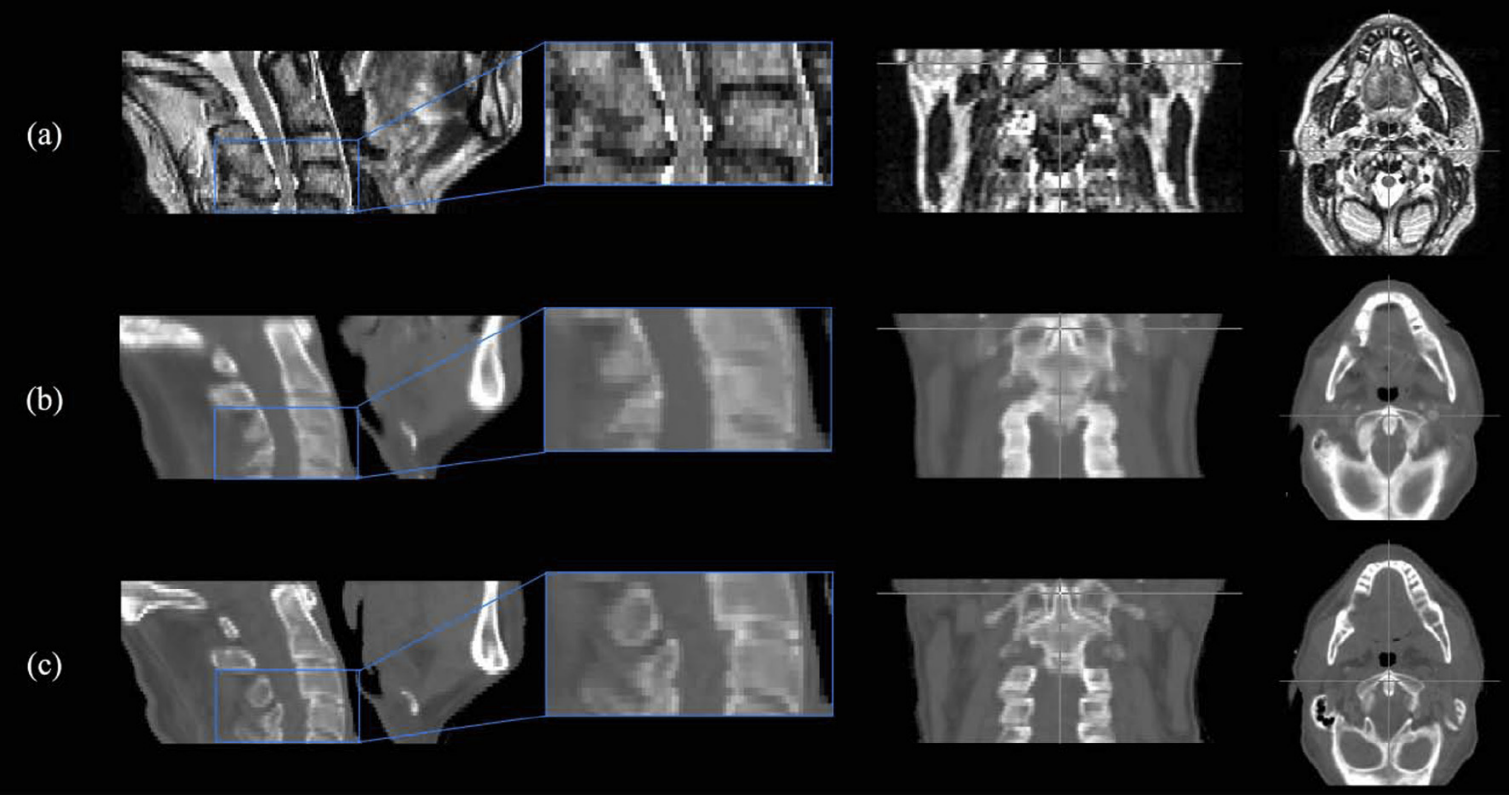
Sagittal, coronal and transverse plane images for a representative H&N patient showing (a) the MRI, (b) the sCT_a_ and (c) the planning CT. MR and sCT_a_ images were non-rigidly aligned to the planning CT for all patients.
